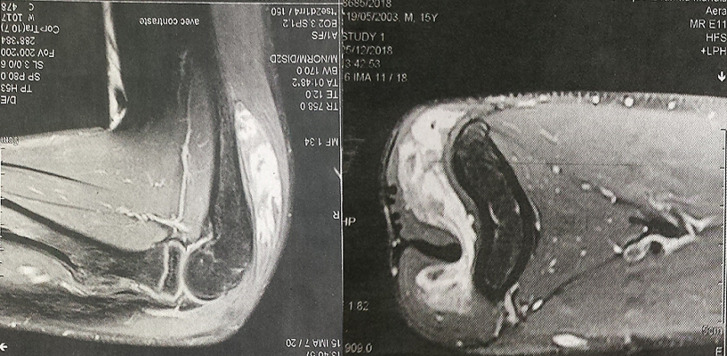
MRI of the elbow showing a hypervascularized mass depending on the triceps
